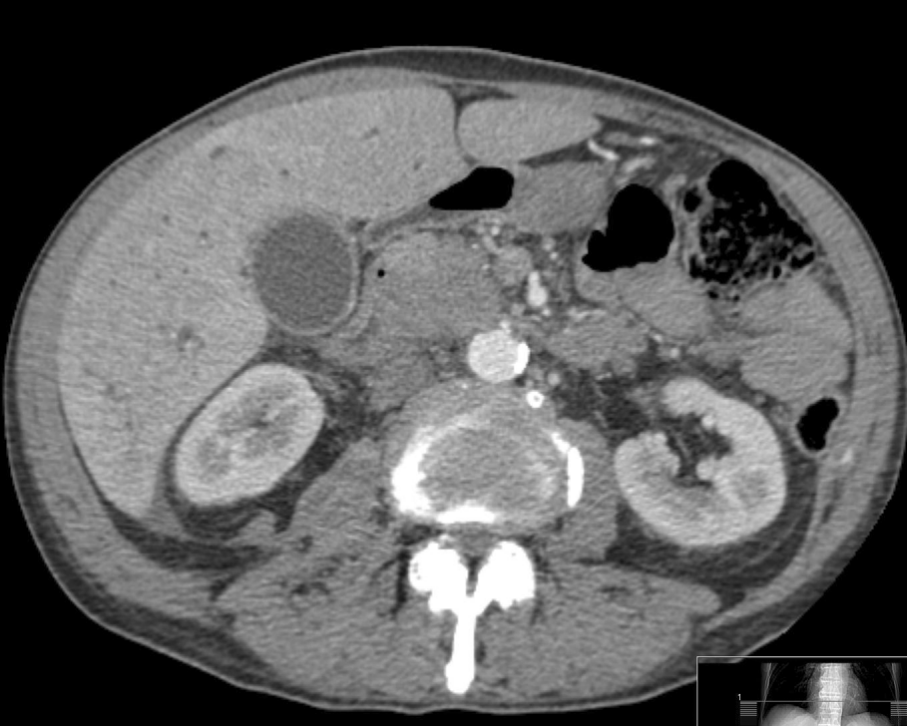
Axial computed tomography image showing a low-density soft-tissue mass in the head of the pancreas, with a clear fat plane between the mass and the superior mesenteric vein.
